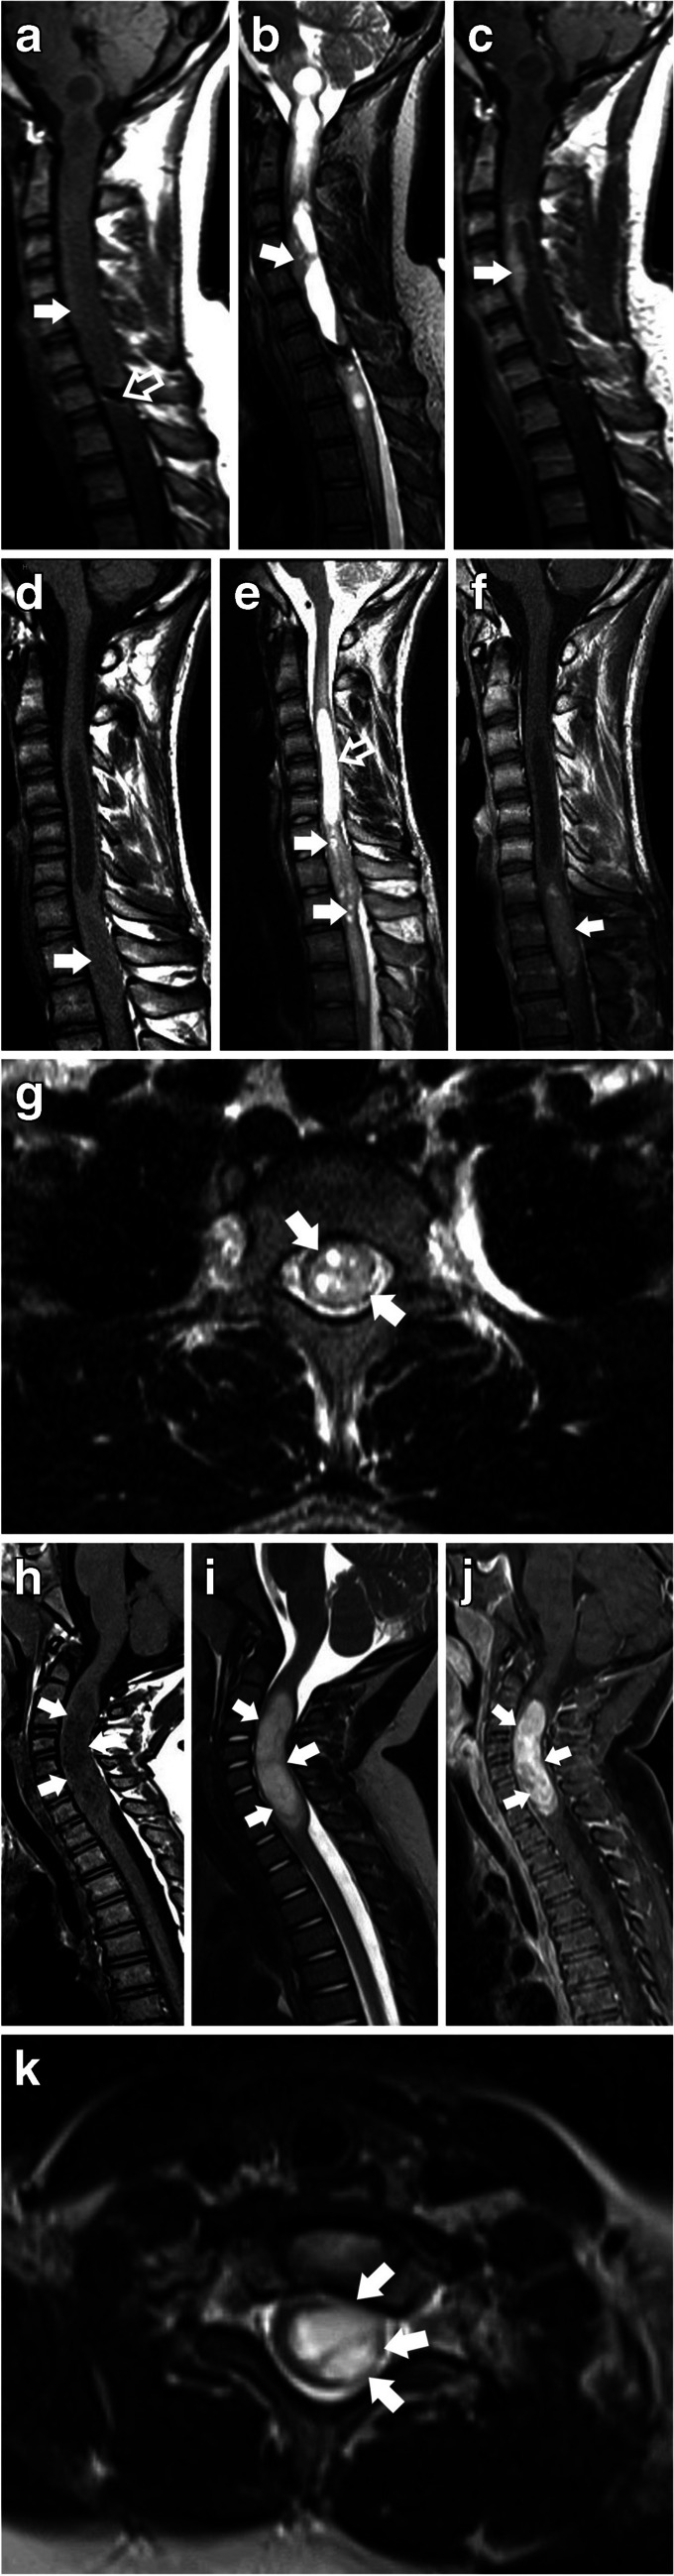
(a - k). Examples of Imaging Features in Astrocytomas vs. Ependymomas. (a – c) Spinal ependymoma WHO 2 in an 18-year-old boy experiencing chronic neck pain, recent onset of weakness in the right hand, decreased deep tendon reflexes in both upper extremities and increased deep tendon reflexes in both lower extremities. a) Sagittal T1 TSE imaging depicts a large, expansile lesion characterized by a predominantly isointense signal (solid white arrows). The mass extends from the pontomedullary junction to the inferior T4 vertebral body. A notable feature is the presence of hemosiderin deposits at the level of C7/T1, indicative of the cap sign (hollow white arrow). b) Sagittal T2 reveals a predominantly cystic lesion with solid tissue seen along the periphery extending from C3-C5 (solid white arrow). c) Sagittal T1 TSE, after contrast administration, results in enhancement of the solid component extending from C3-C5, demonstrating a nodular/mass-like enhancement pattern with ill-defined/shaggy borders (Type 1b) (solid white arrow). (d – g) Spinal ependymoma WHO 2 in a 17-year-old male with 1 week of leg numbness and weakness. d) Sagittal T1 shows an isointense intramedullary expansile lesion extending from C7 to T2 levels and measuring 45mm in length (solid white arrow). e) Sagittal T2 shows multiple intrinsic foci of T2 hyperintensity, representing cystic/necrotic changes within the mass (solid white arrows). There is associated syringomyelia extending from C2 to C6 (hollow white arrow). f) Sagittal T1 TSE after contrast administration shows a nodular/mass-like enhancement pattern with well-defined/crisp borders (type 1a) (solid white arrow). g) Axial T2 shows the mas has a central location within the spinal cord (solid white arrows). (h - k) Pilocytic astrocytoma in a 3-year-old girl with a 5-week history of left arm weakness. h) Sagittal T1 shows a hypointense expansile solid lesion, extending from C2 to C7, measuring 45mm in length (solid white arrows). i) Sagittal T2 shows hyperintense signal of the solid component (solid white arrows). j) Sagittal T1 fat-saturated MR image, after contrast administration, shows a nodular/mass-like enhancement and well-defined/crisp borders (type 1a) (solid white arrows). k) Axial T2 shows a slightly eccentric mass (solid white arrows)
